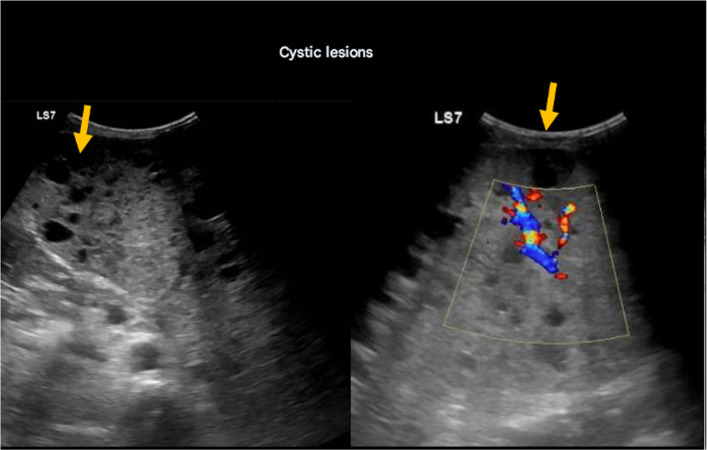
Ultrasound of spleen showing enlargement and multiple cystic lesions
